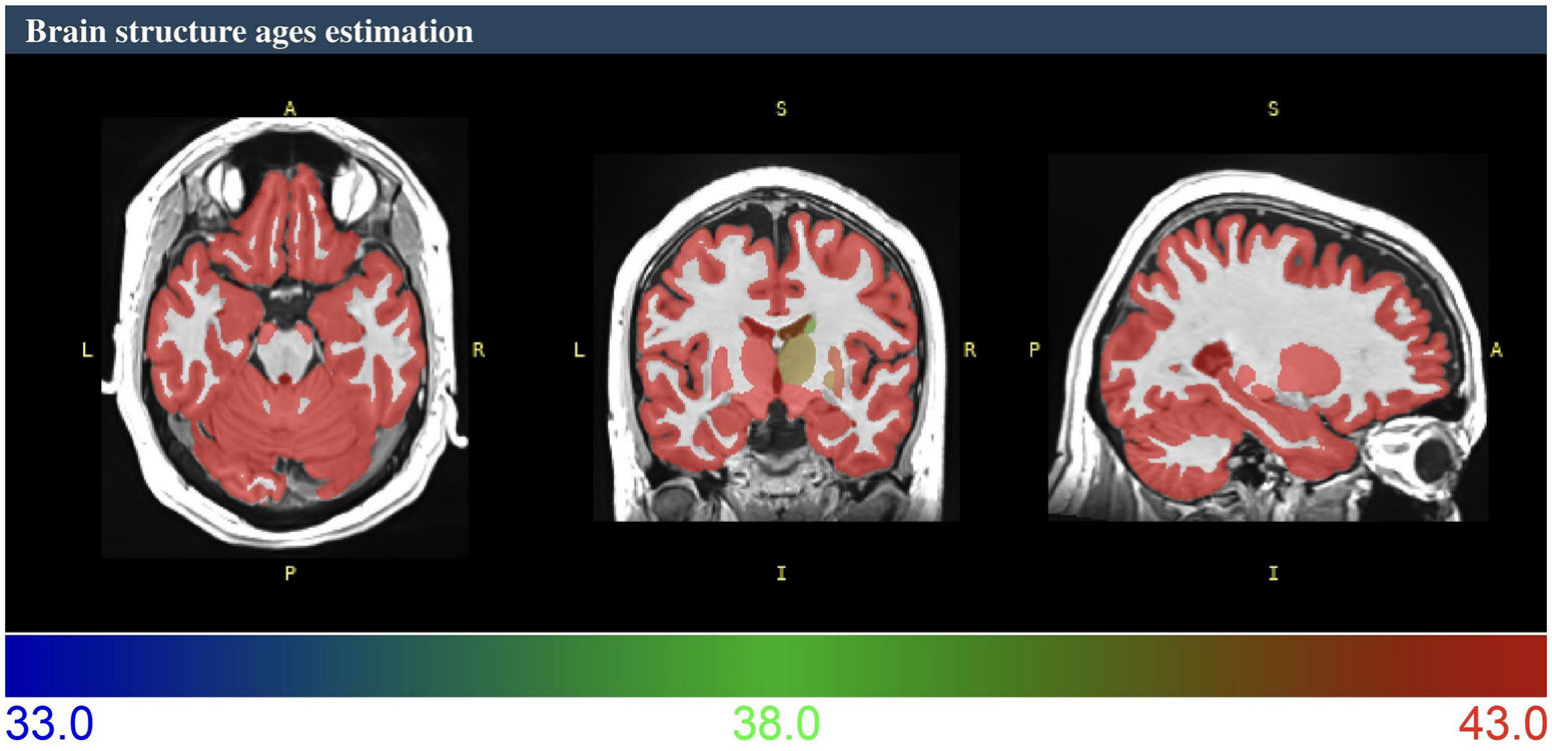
A sample analysis of structural T1‐weighted MRI for brain structure age (BSA) estimation using the volBrain platform (range represents years; the chronological age of this patient was 38 years at baseline).
